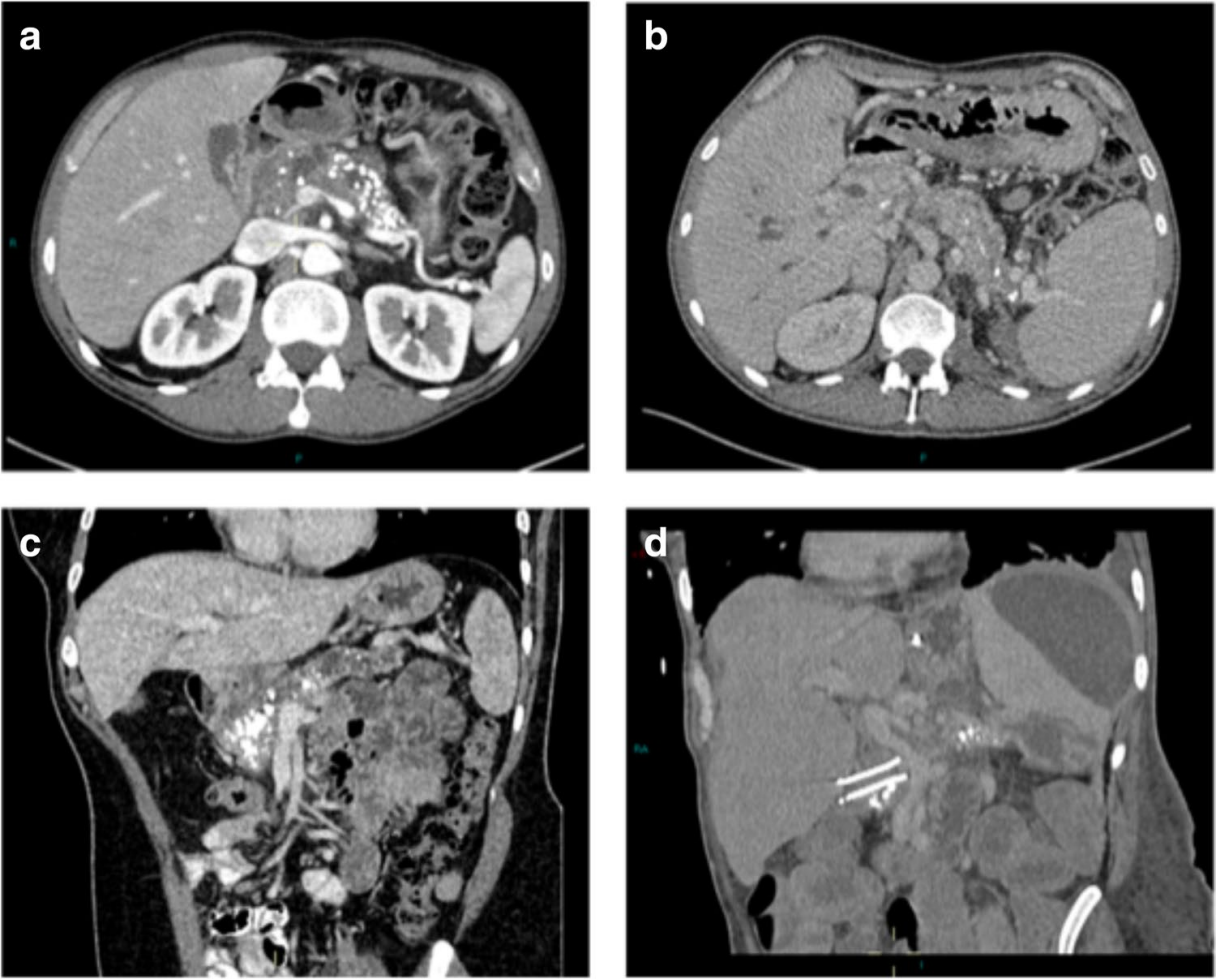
Pre-operative CT images from four different patients. a Parenchymal atrophy and main pancreatic duct dilatation with diffuse parenchymal and ductal calculi. Stenosis of the splenic vein and varices. b Hepatic portal and splenic vein thrombosis, and splenomegaly, with splenic and gastric vein varices. Extra-hepatic bile duct occlusion with intra-hepatic duct dilatation and a previous left nephrectomy. c Pancreatic parenchymal atrophy with diffuse pancreatic parenchymal and ductal calculi and upstream main pancreatic duct dilatation. Splenic and gastric vein varices. d Duodeno-pseudocystic covered stent, non-occlusive hepatic vein thrombus and splenic vein occlusion, upper abdominal varices, splenomegaly with inferior pole infarction and large subcapsular collection. Left-sided pleural effusion
